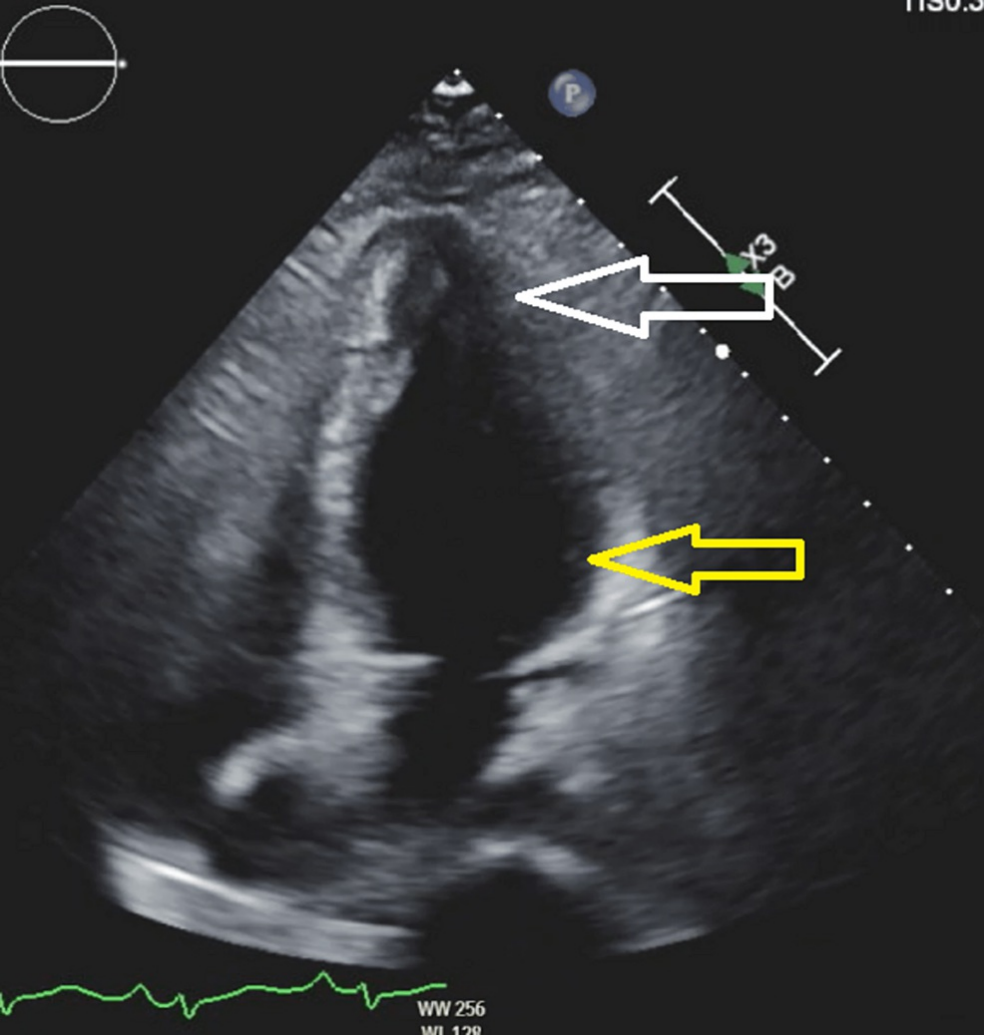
TTE: hyperkinetic apex (white arrow) and hypokinetic base (yellow arrow) of the LV chamber in systole TTE: transthoracic echocardiogram; LV: left ventricle
